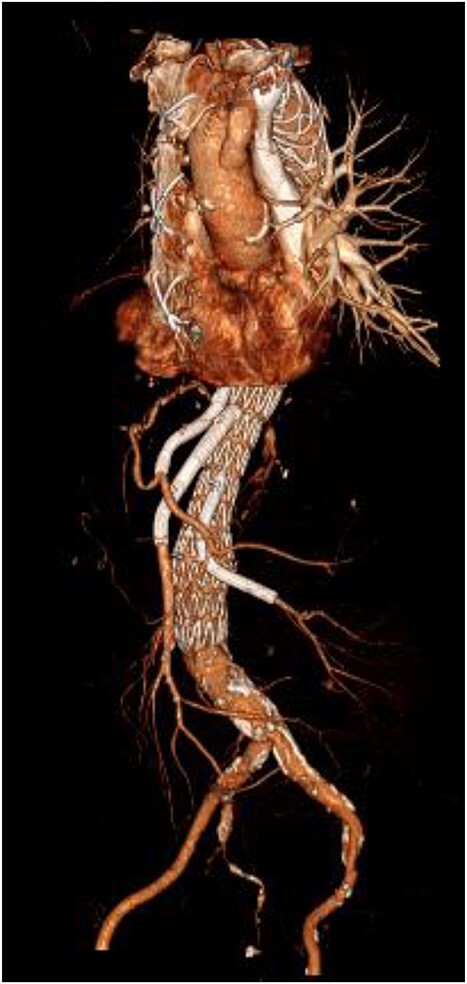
3D reconstructed CT thoracic aorta image showing successful FET implantation and TEVAR.
